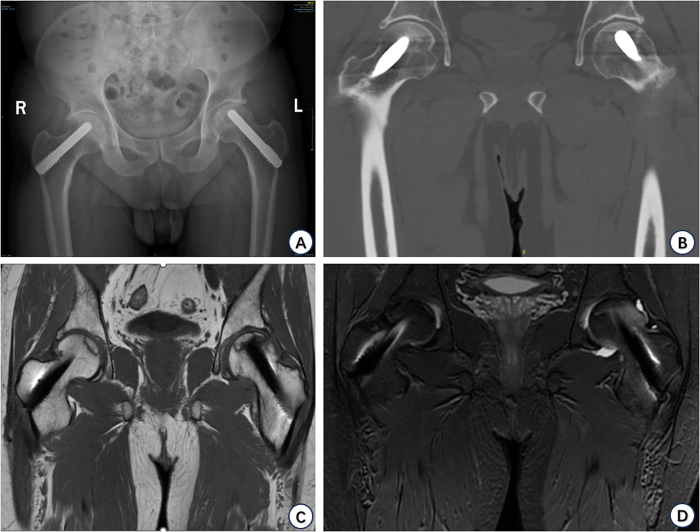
Patient’s preoperative imaging data. (A) Bilateral hip anteroposterior position (avascular necrosis of the femoral head bilaterally after tantalum rod implantation, secondary to osteoarthritis cystic degeneration of the hip joint; internal implants were visible). (B) CT coronal position (subchondral separation of the distal end of the tantalum rod on the left femoral head was visible, suggesting that instability of the femoral head mechanical structure was already present). (C and D) Coronal MRT1 and MRT2 phases (bilateral tantalum). (Bone growth and sclerotic bands are seen around the rods, and bone marrow edema is seen on the lateral side of the left femoral head).
